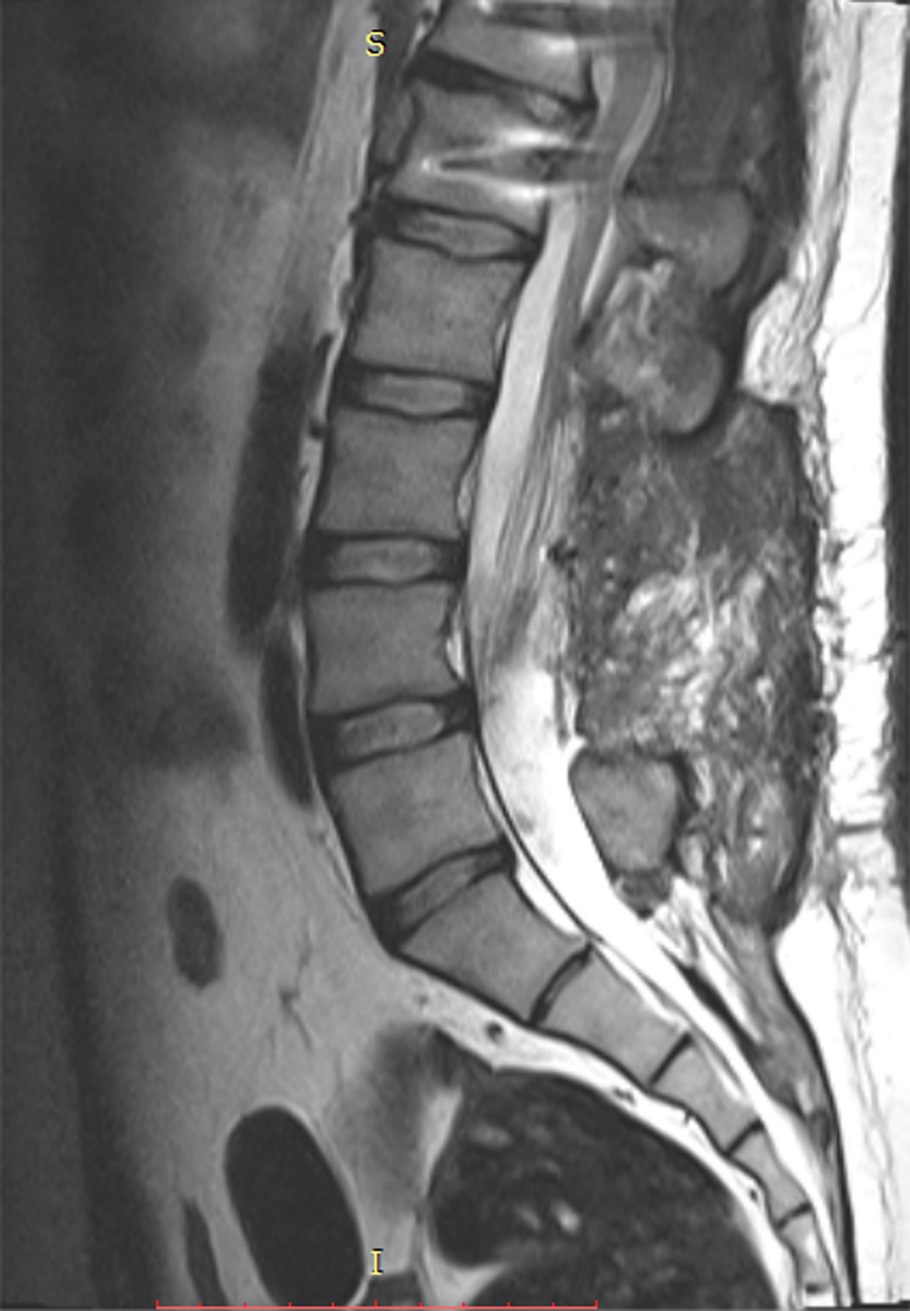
Sagittal T2‐weighted MRI 4 months after surgery shows no evidence of remnant or recurrence.
